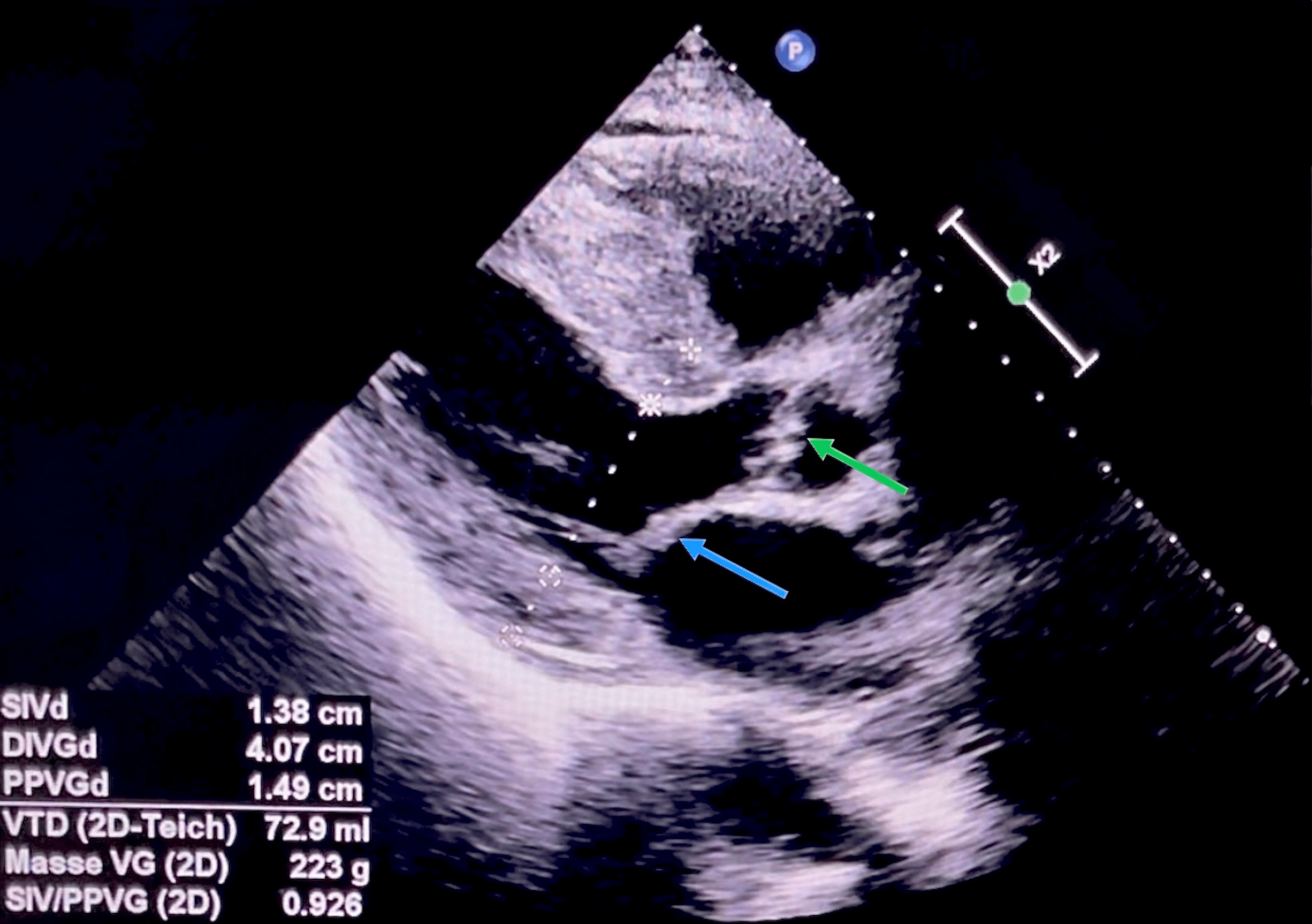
Parasternal view on transthoracic echocardiogram showing concentric LVH, thickened mitral (blue arrow) and aortic (green arrow) valves, and sparkling granular appearance of the myocardium SIVd: interventricular septum thickness at end-diastole (IVSd) = 13.8 mm (normal range (NR): 6-10), DIVGd: left ventricular internal dimension at end-diastole (LVIDd) = 40.7 mm (NR for men: 42-59), PPVG: posterior-wall thickness at end-diastole (PWTd) = 14.9 mm (NR: 6-10), Masse VG: left ventricular mass = 223 g (NR for men: 96-200), corresponding to 132 g/m^2^ (NR for men: 50-115)
